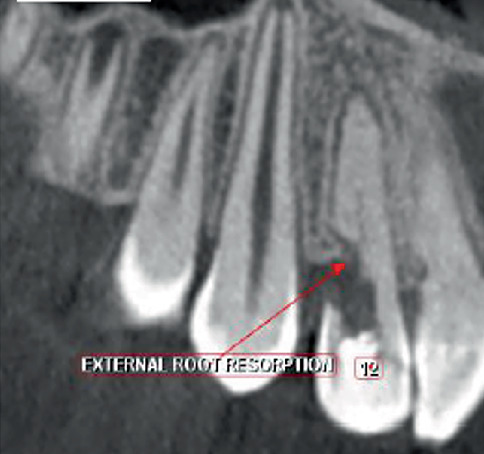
CBCT image showing coronal view of Tooth Number 12 with cervical root resorption and calcified root canal.
